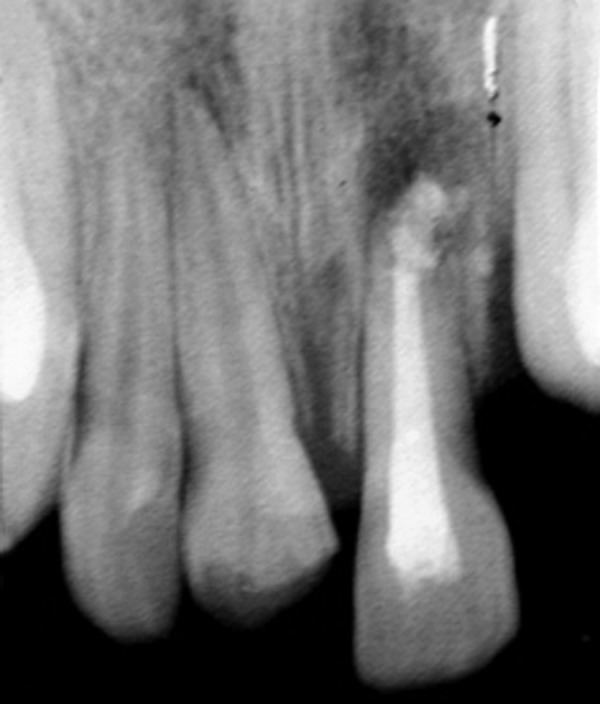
Intraoral periapical radiograph, postsurgery (case 3)
